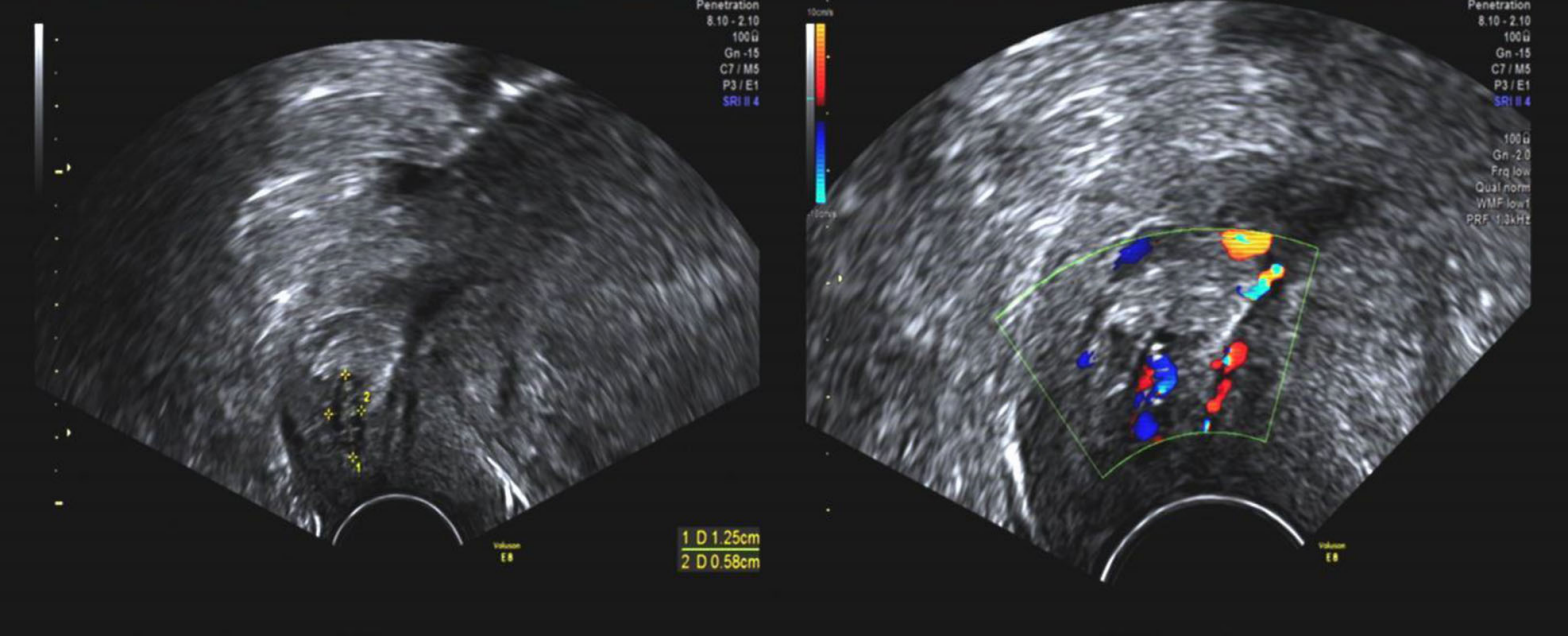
Case 2 ultrasound imaging of cervical pregnancy at the time of diagnosis
